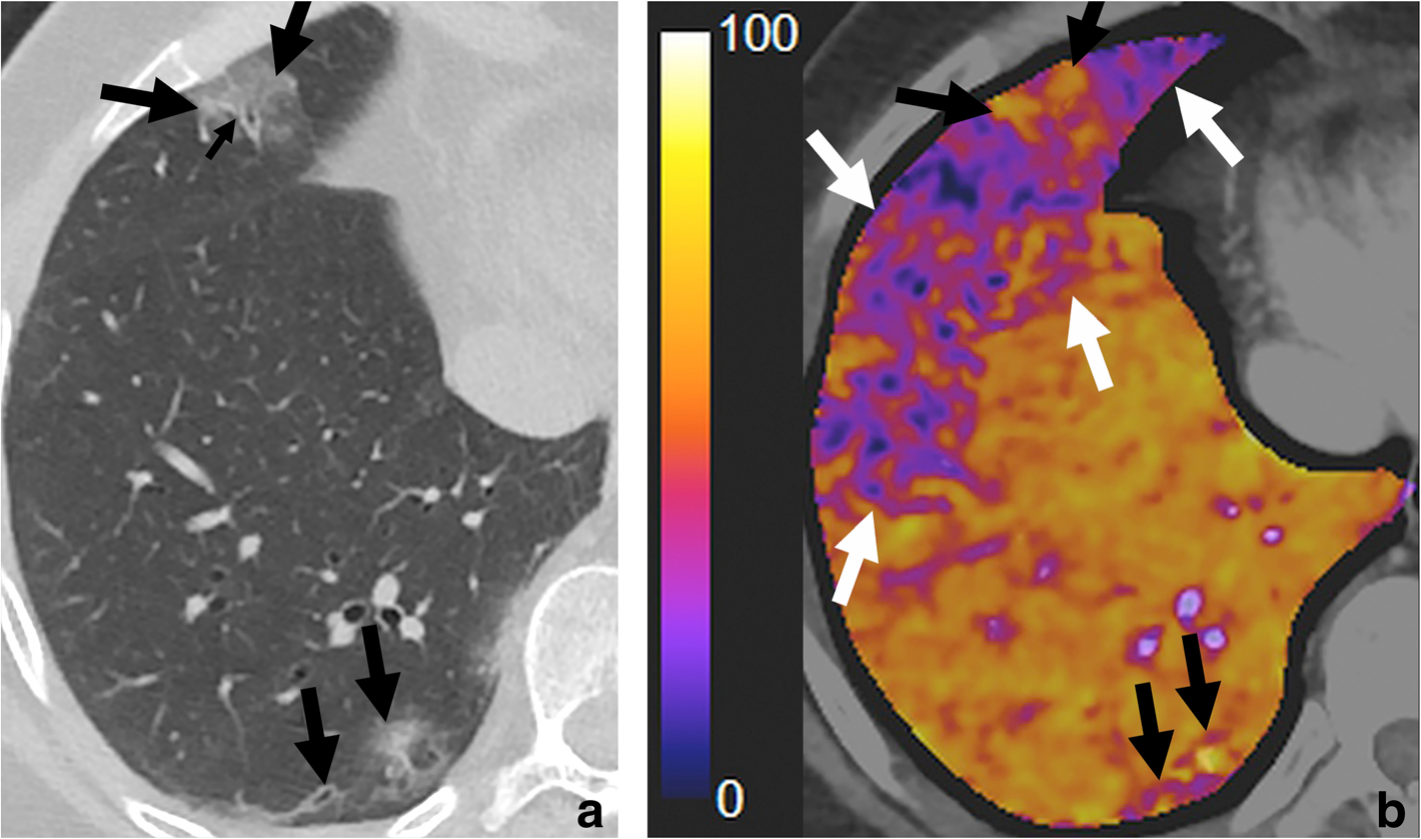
a, b Slight hypoperfusion in the well-aerated lung, hyperemia, and small zones of hypoperfusion in the areas of injured lung. Fifty-nine-year-old male patient, RT-PCR-confirmed COVID-19, 11 days since symptom onset, without hypoxemia, (PaO_2_/FiO_2_) 538, d-dimer 340 ng/mL. There are isolated foci of ground-glass opacities associated with septal thickening, with a predominantly subpleural distribution, which correlate with areas of hyperemia (middle lobe) and small zones of hypoperfusion (lower right lobe) in subtraction CT iodine maps (large black arrows). There is an evident area of hypoperfusion in the middle lobe and lower right lobe (white arrows) that correlates with the apparently normal lung parenchyma in conventional chest CT images. The conventional CT image also shows pulmonary arterial vascular dilatation in the periphery of the ground-glass opacity in the middle lobe (small black arrow). These slight perfusion abnormalities do not impact the PaFi ratio. The ground-glass opacity in the lower right lobe shows slight peripheral hypoperfusion, probably due to compensatory vasoconstriction, an expected regulatory mechanism when vasoplegia is not fully established
